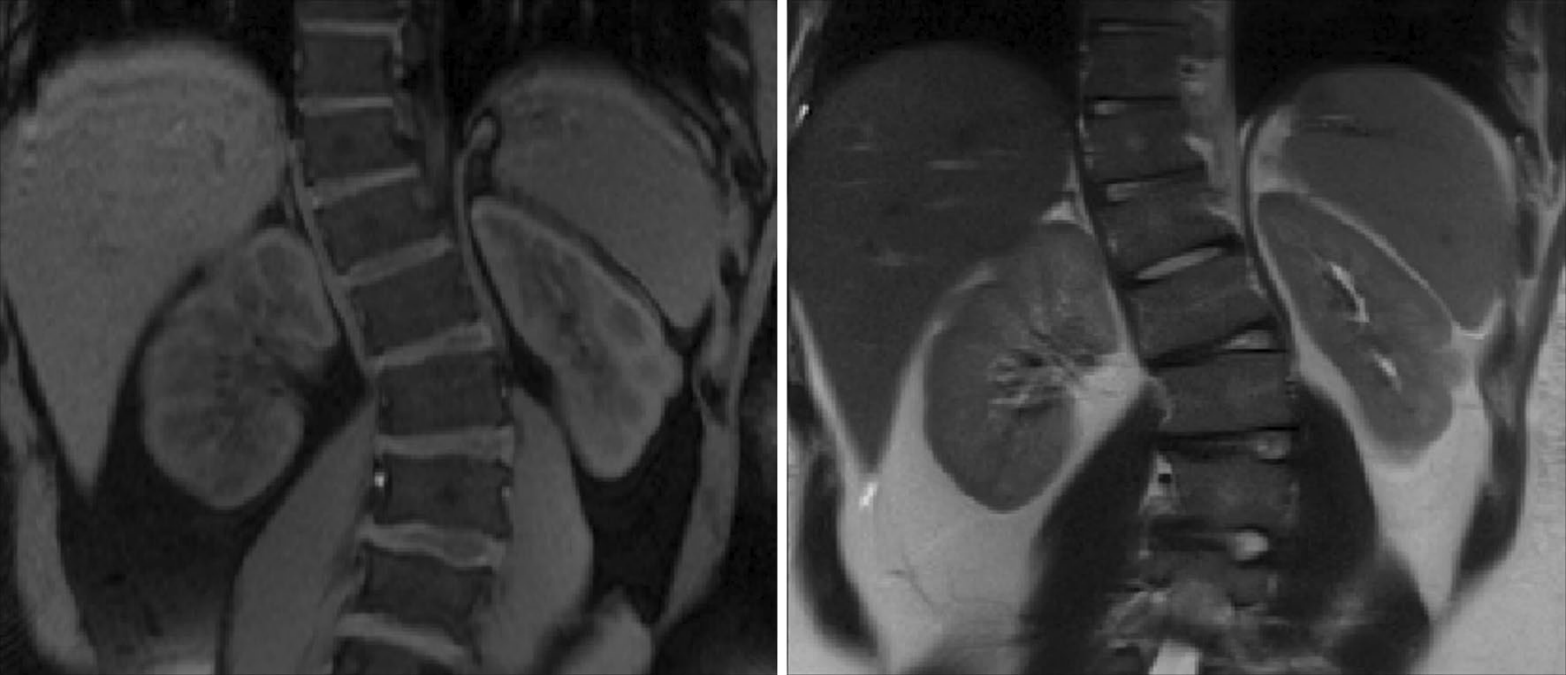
T1- (left) and T2-weighted (right) MRI images of a BBS subject included in the present study. The morphological analysis shows fetal lobulations at the inferior pole of the left kidney.
